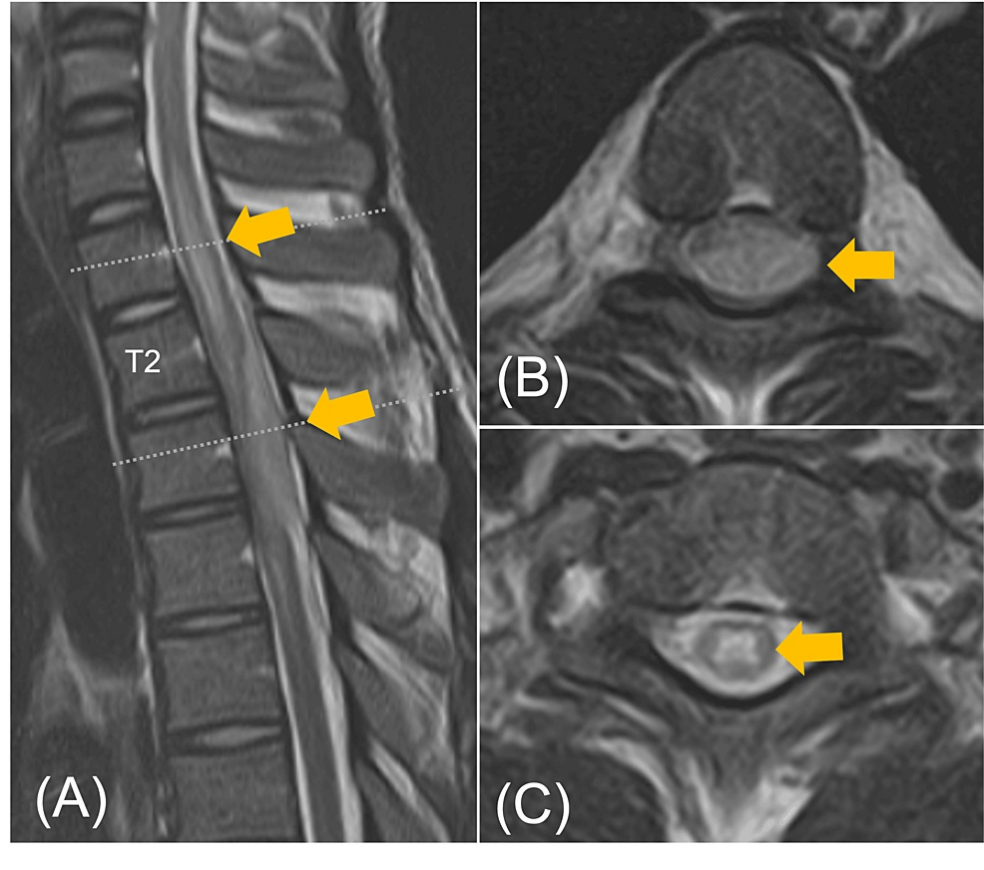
Magnetic resonance imaging (MRI) of the thoracic spine (A) A sagittal T2-weighted magnetic resonance imaging of the spine demonstrates an intradural extramedullary lesion of hyperintense signal intensity, compressing the spinal cord at the T2-T4 vertebral levels. (B) An axial T2-weighted magnetic resonance imaging at the T3 level revealed the presence of an intradural extramedullary lesion occupying the dorsal aspect of the spinal cord. (C) An axial T2-weighted magnetic resonance imaging at the T1 level revealed changes in intramedullary signal intensity surrounding an intradural extramedullary lesion.
